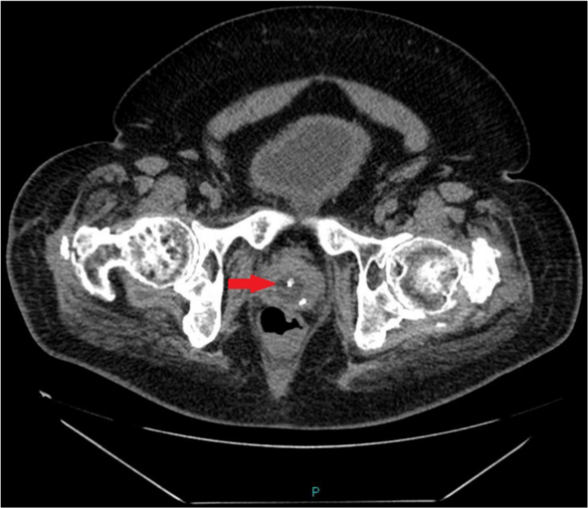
Axial scan of CT of pelvis performed ten days later: Neither the catheter nor balloon of Foley catheter can be seen inside the urinary bladder. The balloon of Foley catheter (arrow) is located in dilated prostate-membranous urethra.
